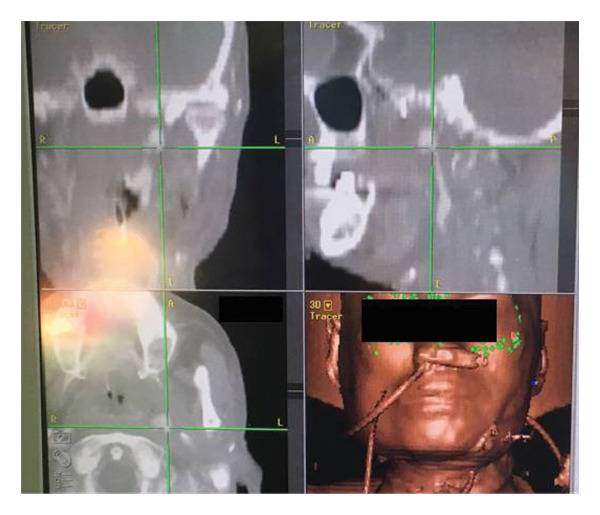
Intraoperative navigation system used to assist in surgical ligation of the pseudoaneurysm via an infratemporal approach during the second episode of carotid blowout syndrome. Multiplanar CT navigation images are shown in counterclockwise order from the upper right to the lower left (sagittal, coronal, and axial views), with three‐dimensional surface registration in the lower right panel. The green crosshairs in all planes are centered on the target pseudoaneurysm, demonstrating precise localization prior to ligation. (In the lower right panel, the rope‐like structures visible on the patient’s face represent the surgical navigation reference markers and registration cables, while the green dots indicate the tracked fiducial points used by the navigation system for spatial registration).
